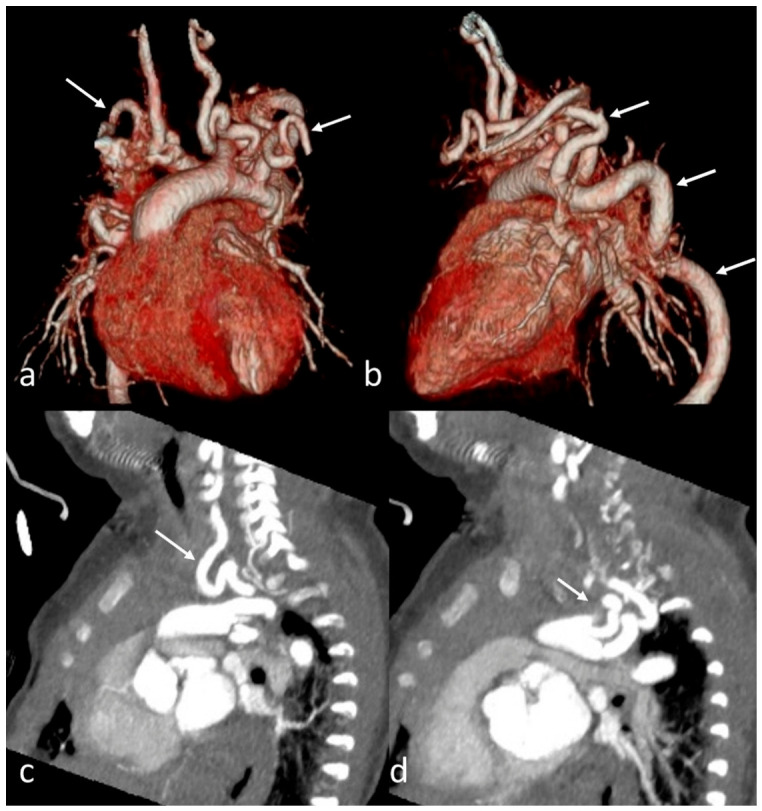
Cardiovascular computed tomography angiography findings at 1 month of age. Cardiac volume-rendered computed tomography angiography images demonstrate severe tortuosity of the aorta (a,b). Sagittal oblique contrast-enhanced computed tomography images show tortuosity of the right brachiocephalic artery, as well as the right and left common carotid and subclavian arteries (c,d).
